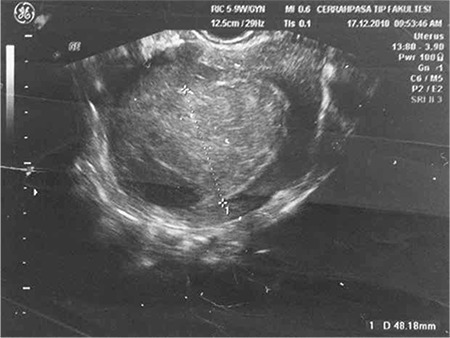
Ultrasound image of the uterine mass
